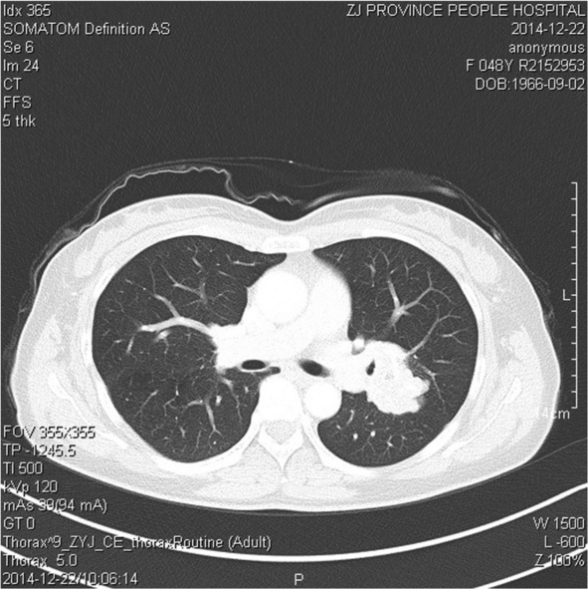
Radiology of ASPS of the lung. Computed tomograph (CT) scan demonstrated a well-demarcated, lobulated, heterogeneously enhanced mass located at the level of hilum of left lung
